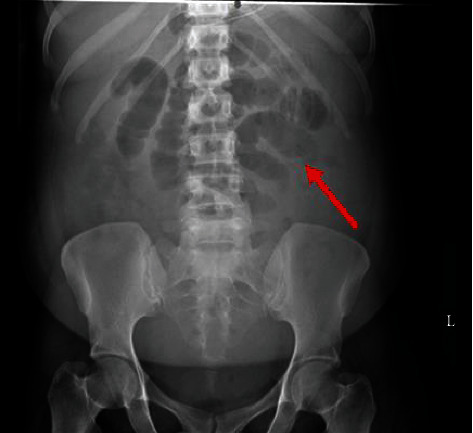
2-view abdominal X-ray showing dilated loops of small bowel.
